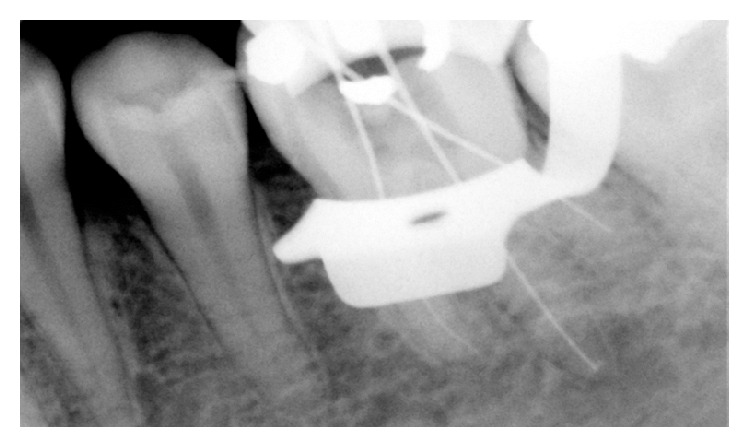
Radiograph of working length with three K-files in MB, DB, and DL root canals.
